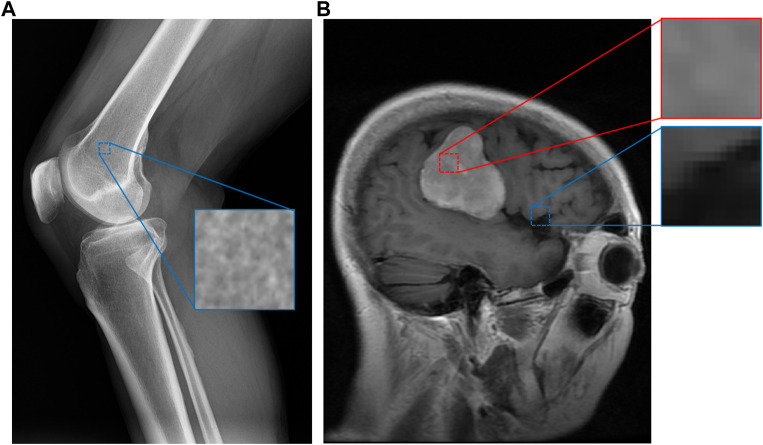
An example of image patches taken from a knee X-ray (A) and a brain MRI with a tumor (B) (Cheng, 2017). Although some categories, such as bone, have apparent material distinctions, others such as tumor (in red) and healthy (in blue) brain tissue may not have obvious material differences. However, our system can still discern these differences since the images are expertly labeled, while assigning smaller perceptual distances (similarity) between pairs of categories more similar to each other.
